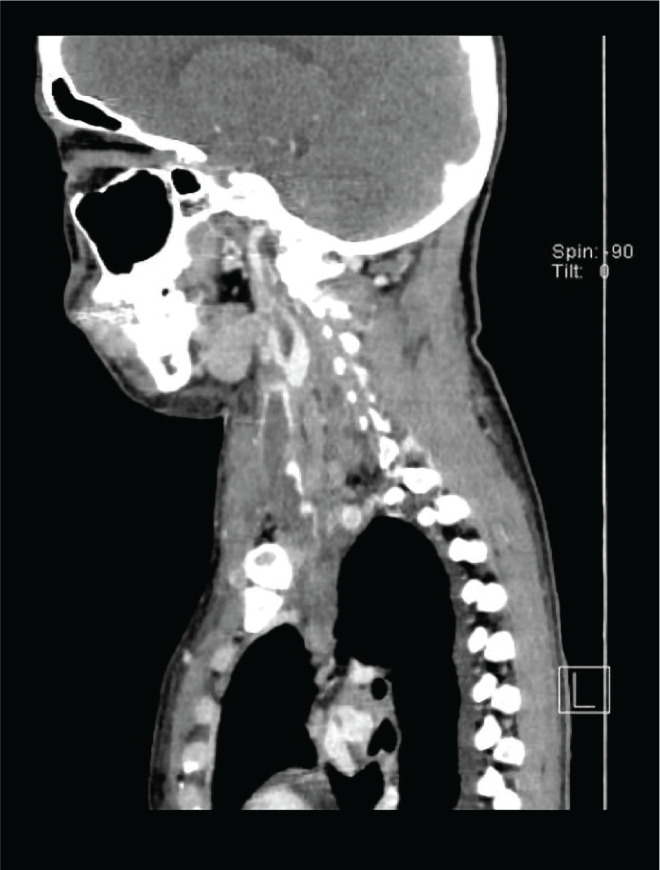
CT scan of the neck (sagittal view): extensive thrombosis of the left internal jugular vein.
